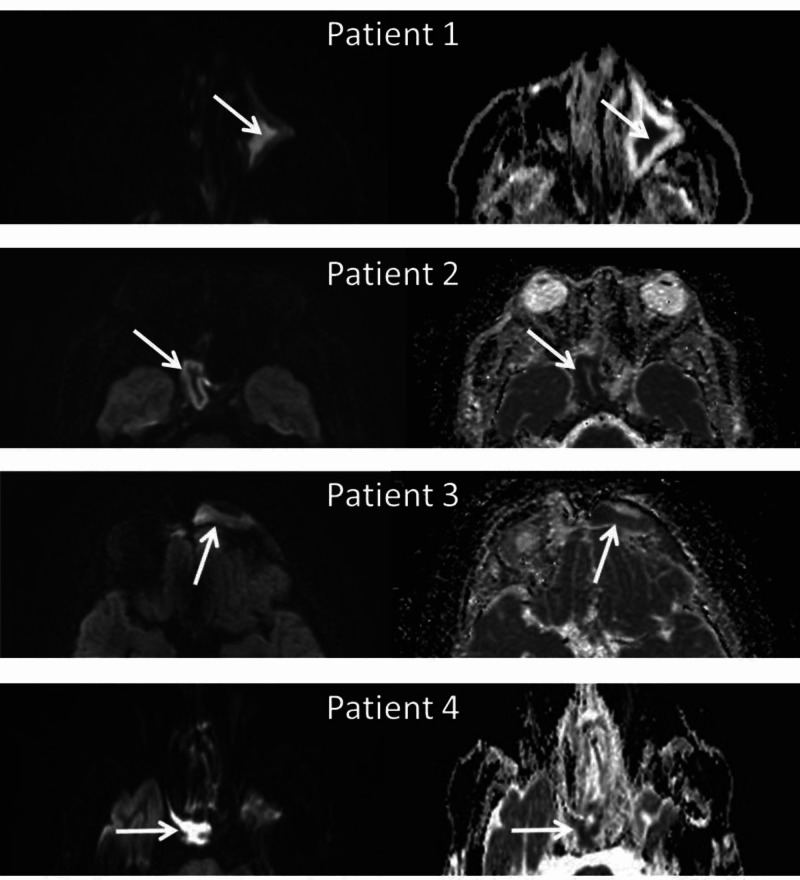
Diffusion-weighted imaging (DWI) magnetic resonance imaging (MRI) in patients with acute bacterial rhinosinusitis Diffusion restriction in culture-positive acute sinusitis (diffusion-weighted image on the left and apparent diffusion coefficient map on the right, arrows point to restricted diffusion).
